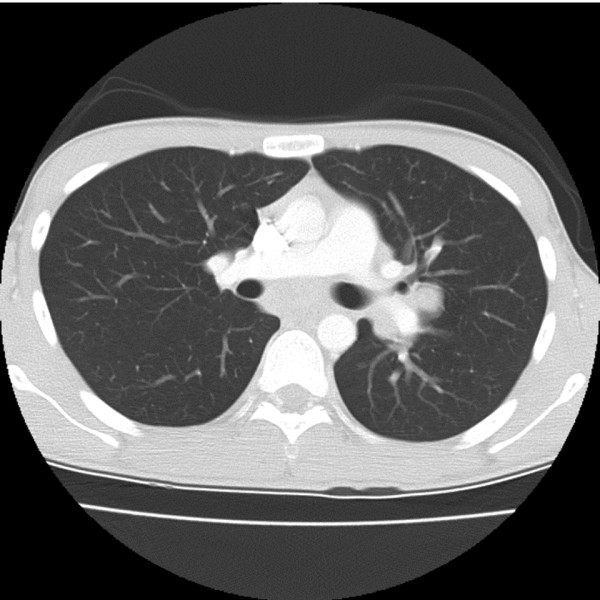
CT scan of his lungs, showing a large central space occupying lesion surrounding both main bronchi.
